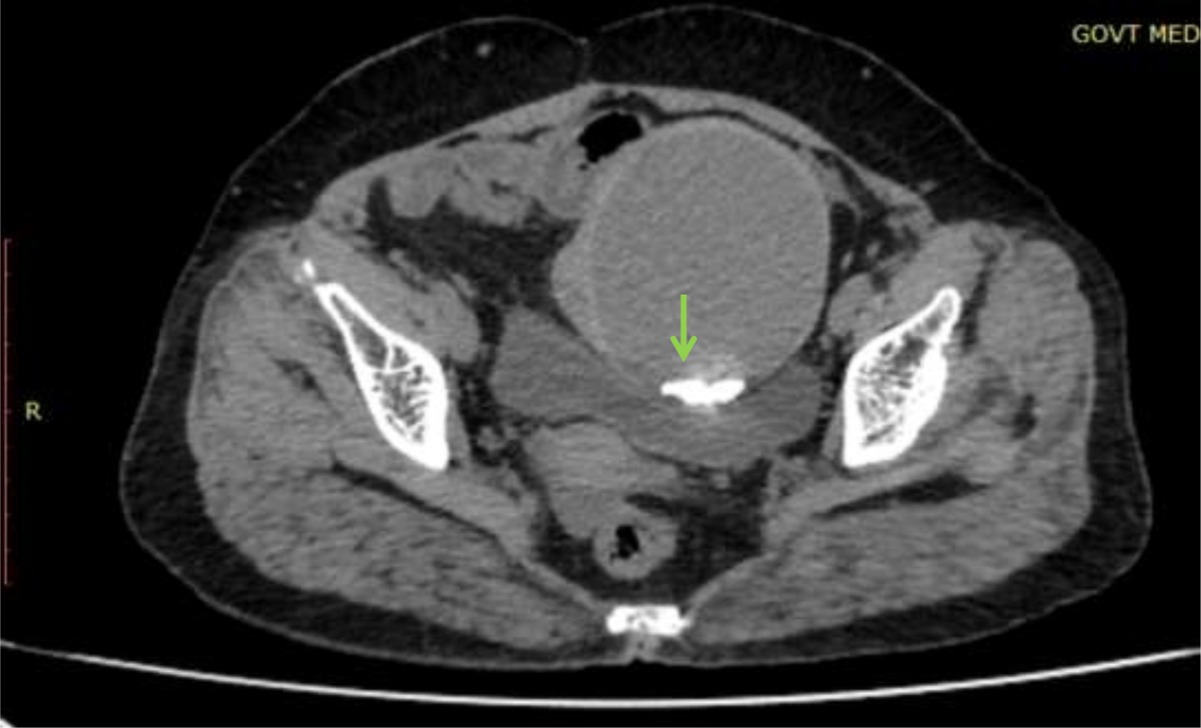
Axial CT images show chunky calcifications in the posterior aspect of the lesion. No septations or solid areas noted within the lesion (green arrow).
